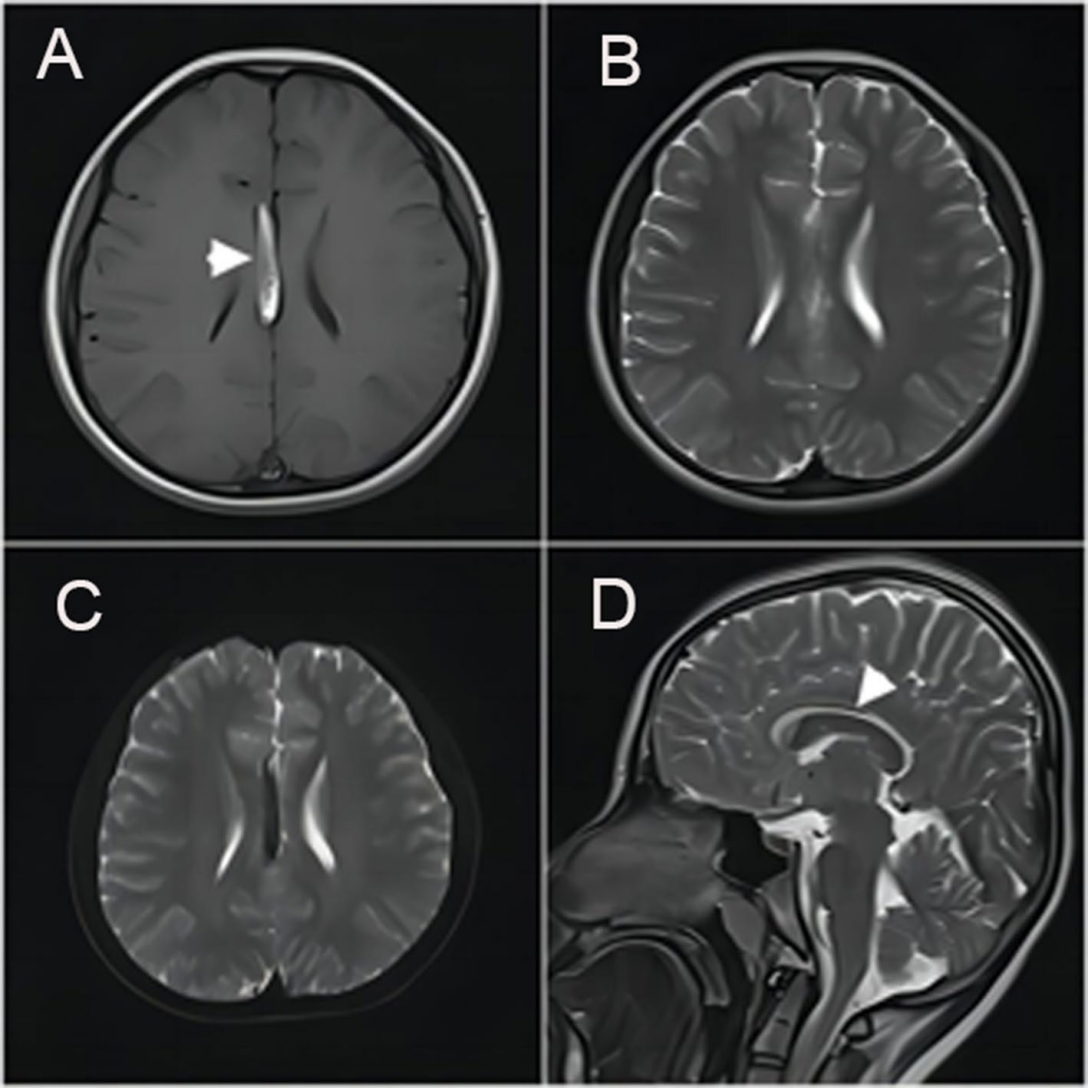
A, B are MRI scanning axial T1 and T2 images, respectively, which show parallel separation of bilateral lateral ventricles, widening of the spacing between the bodies of bilateral lateral ventricles, and a lipoma (white arrowhead) at the lower margin of the cerebral falx, with a size of about 40mm*9mm, with high signal in T1 and T2. C is an axial DWI image; the lesion is seen without diffusion restriction. D is a sagittal T2 image; the septum pellucidum is not closed, and the corpus callosum is thinned in the sagittal position (white arrow)
